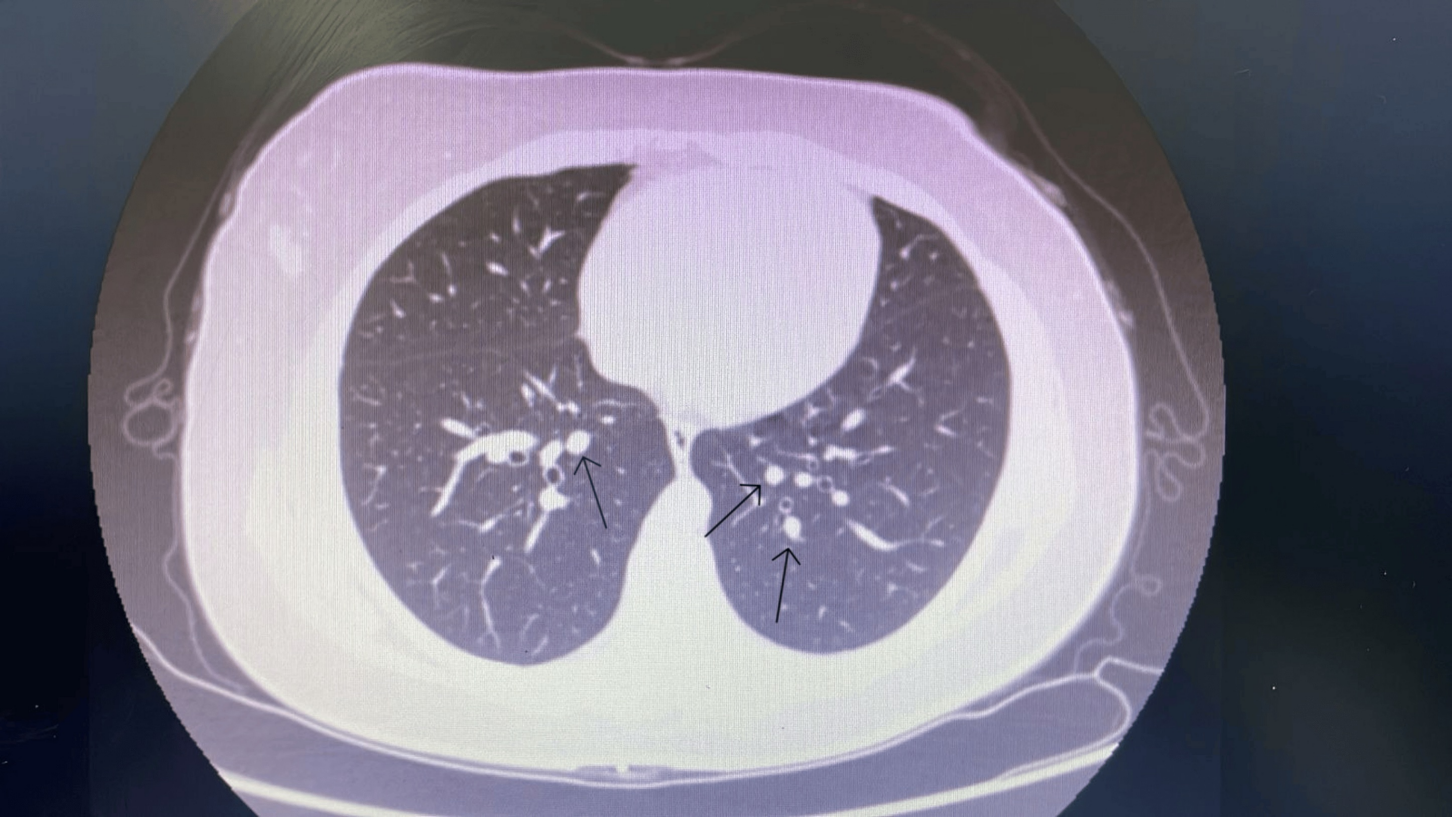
Axial contrast-enhanced CT of the chest demonstrating two subcentimeter pulmonary nodules. A 3.2 mm nodule is seen in the left lower lobe (black arrow), and a 3 mm subpleural nodule is noted in the right middle lobe (black arrow). These indeterminate nodules were recommended for interval surveillance given the patient’s new diagnosis of a high-grade small round-cell tumor
